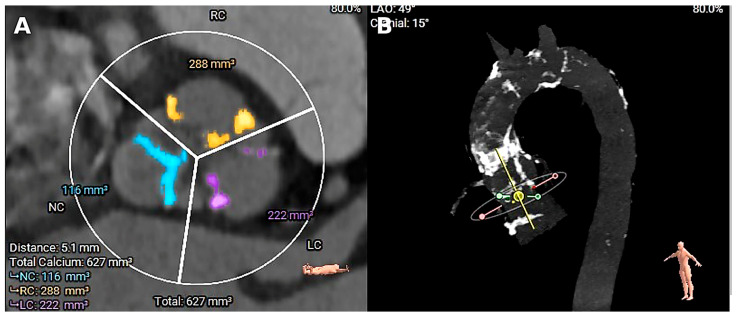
Computed tomography characterization of porcelain aorta and calcific burden relevant to procedural risk. (A) Short-axis multislice computed tomography (“hockey-puck”) view of the aortic valve demonstrating asymmetric leaflet calcification with quantified calcium burden relevant to embolic risk and transcatheter valve deployment. (B) Three-dimensional volume-rendered computed tomography image showing extensive, circumferential calcification of the ascending aorta and proximal aortic arch, consistent with a porcelain aorta and highlighting the prohibitive risk of surgical aortic manipulation.
